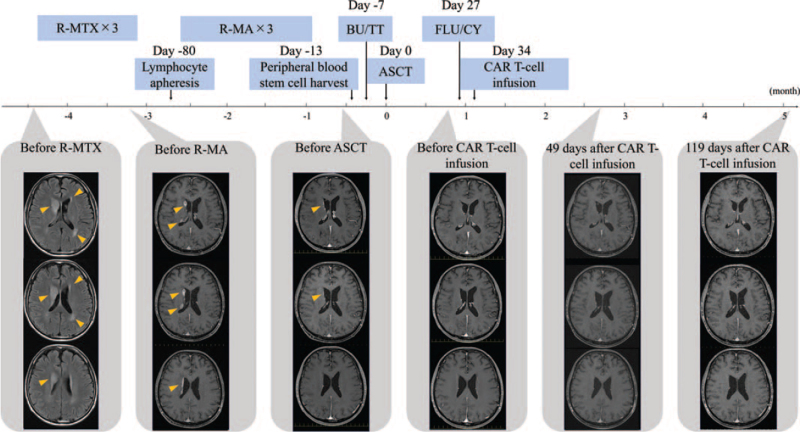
Clinical course: The patient received R-MTX (rituximab and methotrexate) and R-MA (rituximab, methotrexate, and cytarabine) after a central nervous system (CNS) relapse. He received BuTT (busulfan and thiotepa) as the conditioning regimen followed by ASCT (autologous stem cell transplantation). Posttransplantation, he received lymphodepleting chemotherapy consisting of FLU/CY (fludarabine and cyclophosphamide) and an infusion of anti-CD19 CAR (chimeric antigen receptor) T-cells. Brain MRI after the CAR-T cell infusion demonstrated resolution of the CNS lesions, indicating a complete response. The arrowheads show the presence of lymphoma lesions in the brain. MRI = magnetic resonance imaging.
